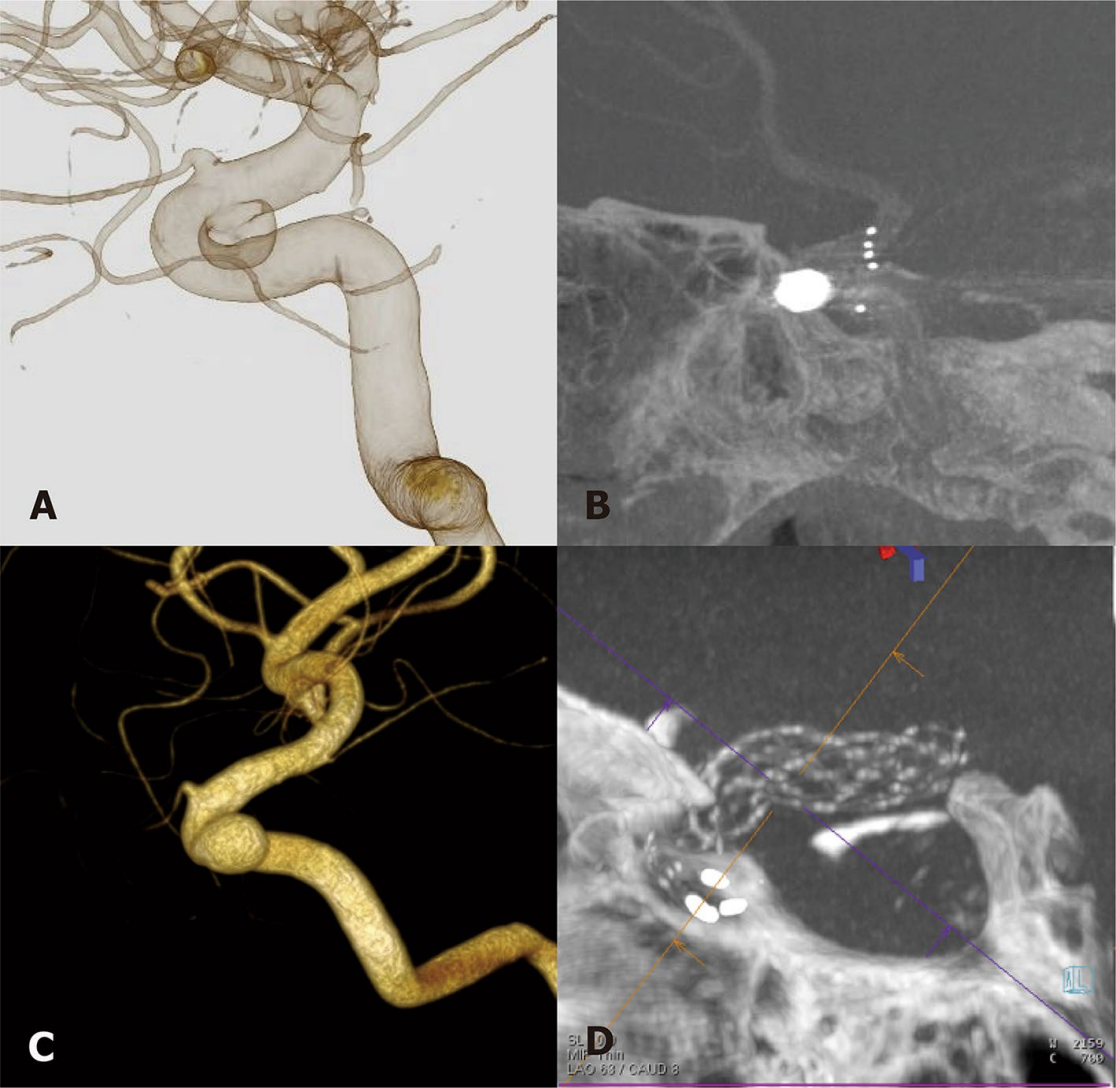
Two representative cases demonstrate successful deployments of the Alpha stents for treating ICA paraclinoid aneurysms. (A) and (C) 3-dimensional rotational angiograms of right ICA paraclinoid aneurysms. (B) and (D) Flat-panel CT images depict good wall apposition of the stents in the anterior genu of the ICA.
